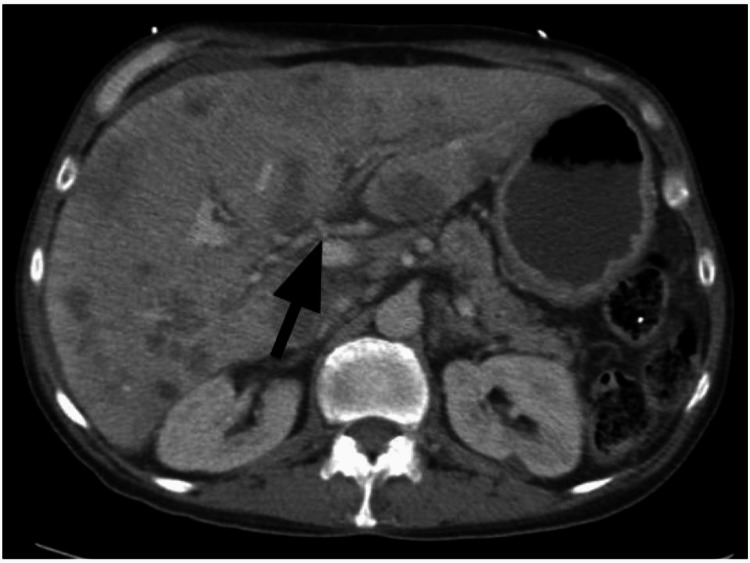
The computed tomography (CT) abdomen and pelvis with contrast revealed a partial left portal vein filling defect suggestive of thrombosis(black arrow).
